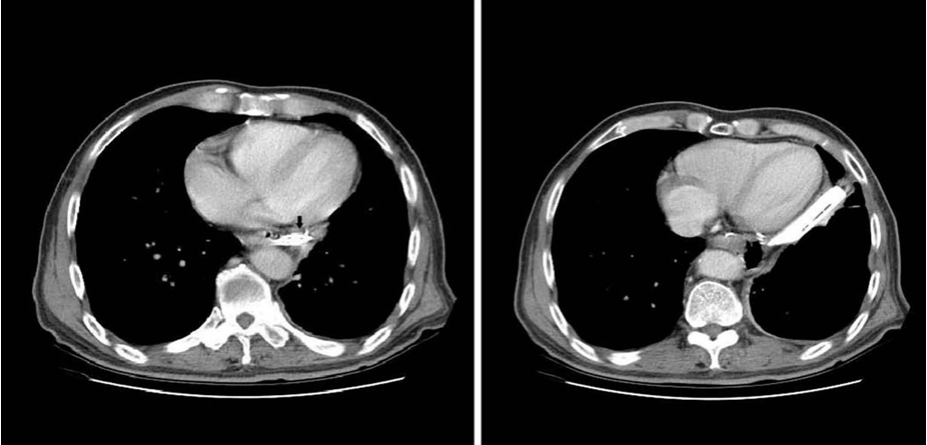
Computed tomography indicates the possibility of drain tube migration into the esophagus (arrow).
